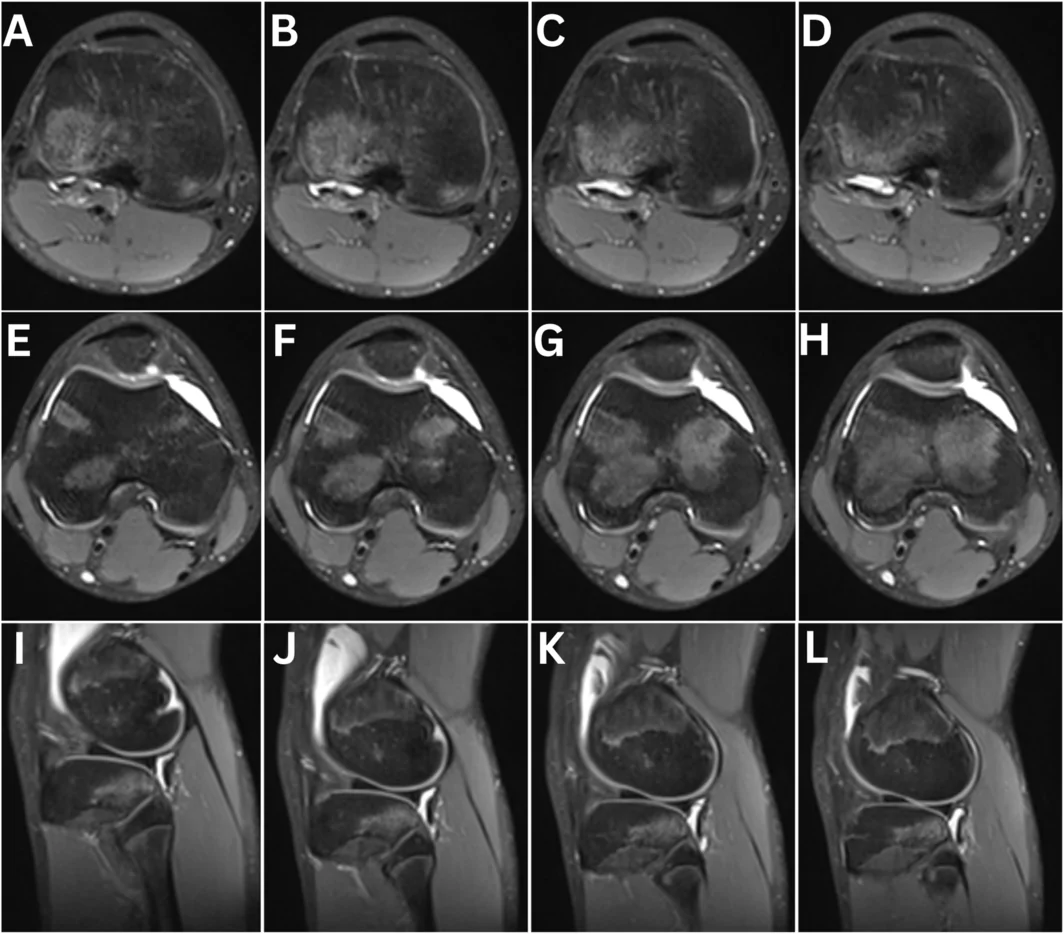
Initial axial MRI showing pivot‐shift bone bruising. Fat‐suppressed fluid‐sensitive images demonstrate patchy bone marrow edema in the posterolateral tibial plateau, extending into the proximal tibial metaphysis (A–D), and corresponding edema in the femoral condyles, particularly the lateral femoral condyle (E–H). Additional sagittal images (I–L) demonstrate the sagittal distribution of the lateral femoral condyle bone contusion.
